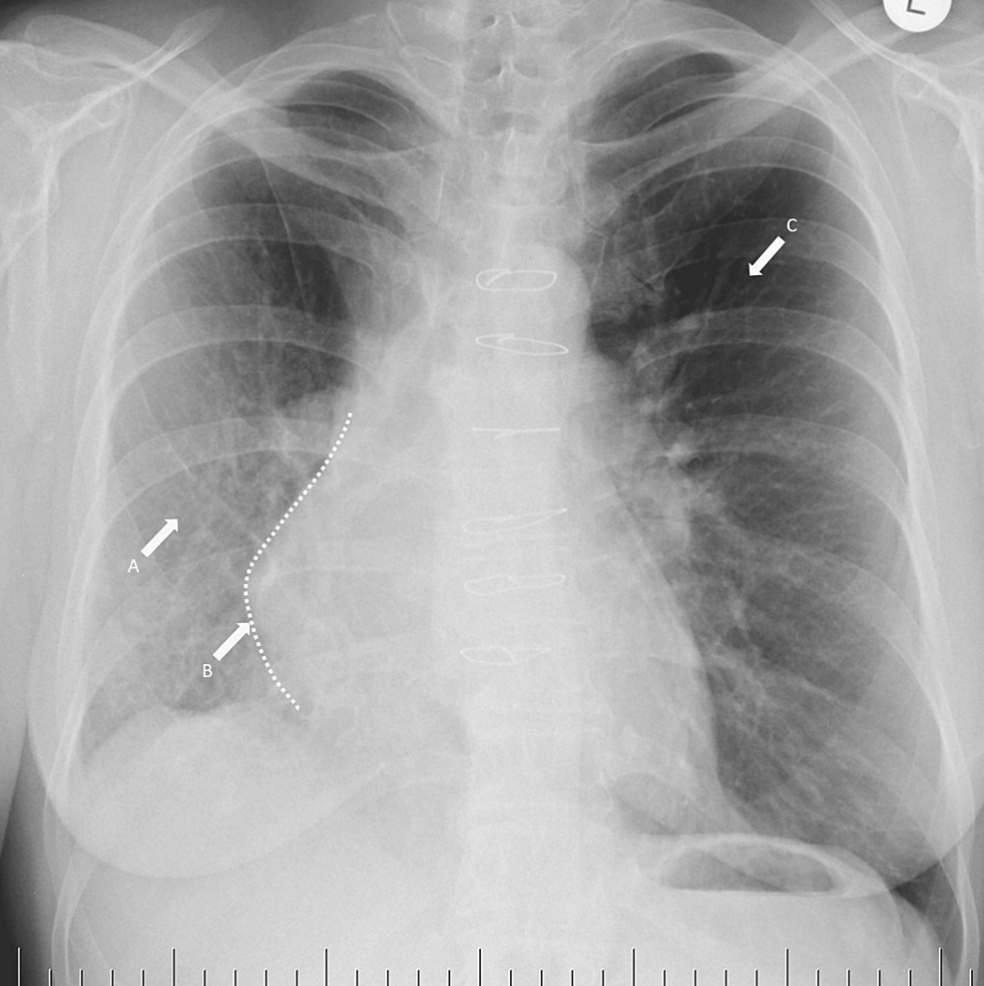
Chest radiograph. Decreased transparency of (A) the right lower lobe, (B) ipsilateral mediastinal shift, and (C) relative hyperlucency of the left upper lobe.
